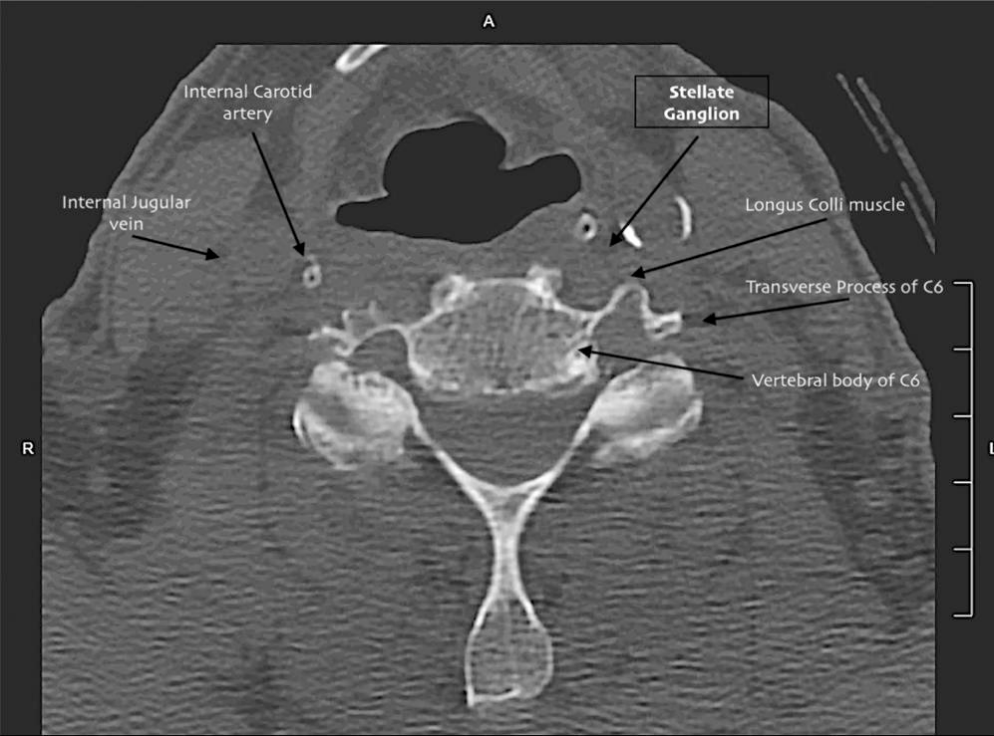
Computed tomography scan cross section of fascial layer of neck. The usual target of stellate ganglion block is the anterior plane of longus colli muscle. Our modified approach to stellate ganglion block takes as target the transverse process of vertebral bone C6.
